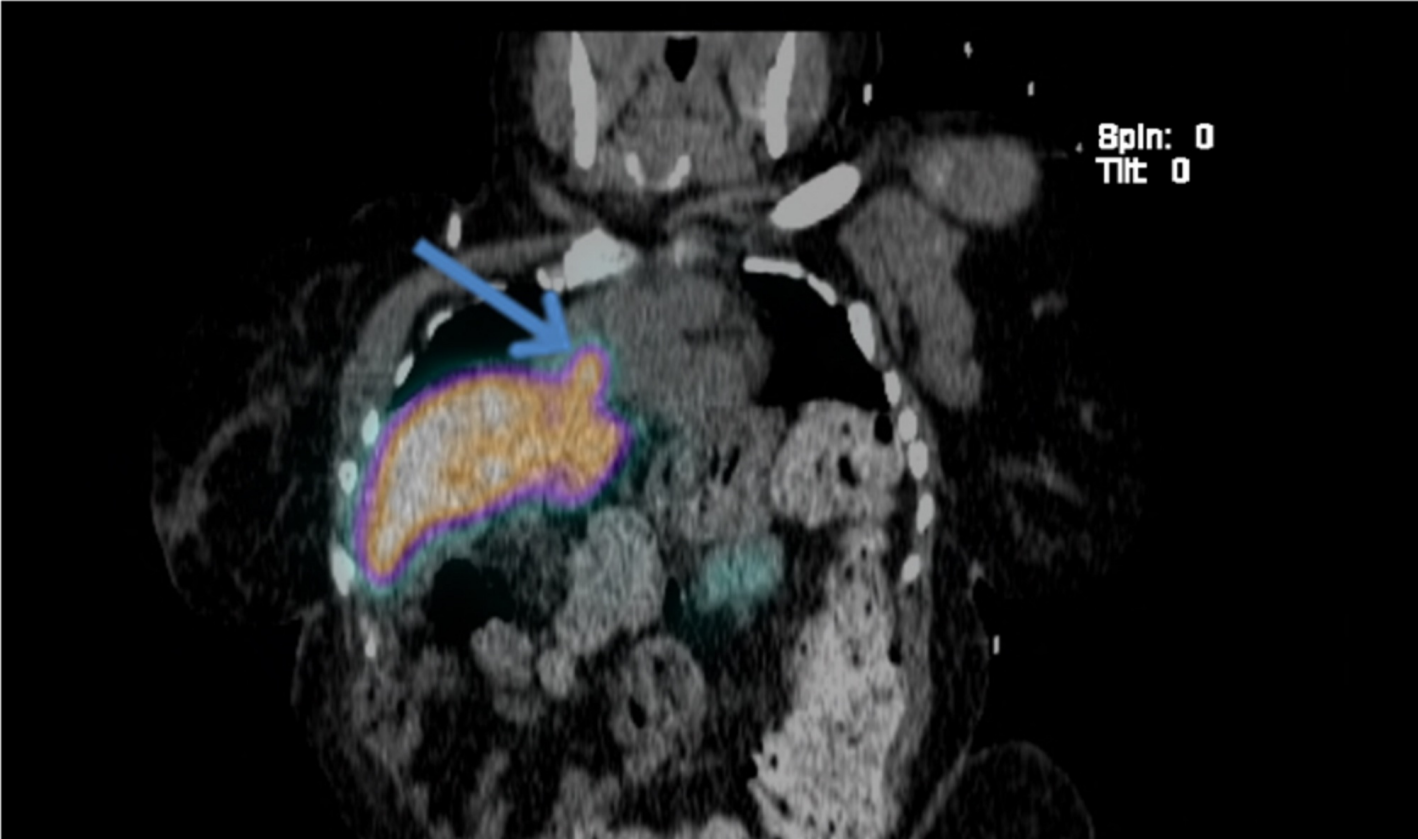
99m Tc-sulfur colloid SPECT/CT showing the liver and liver hernia uptake SPECT/CT: single photon emission computed tomography/computed tomography
